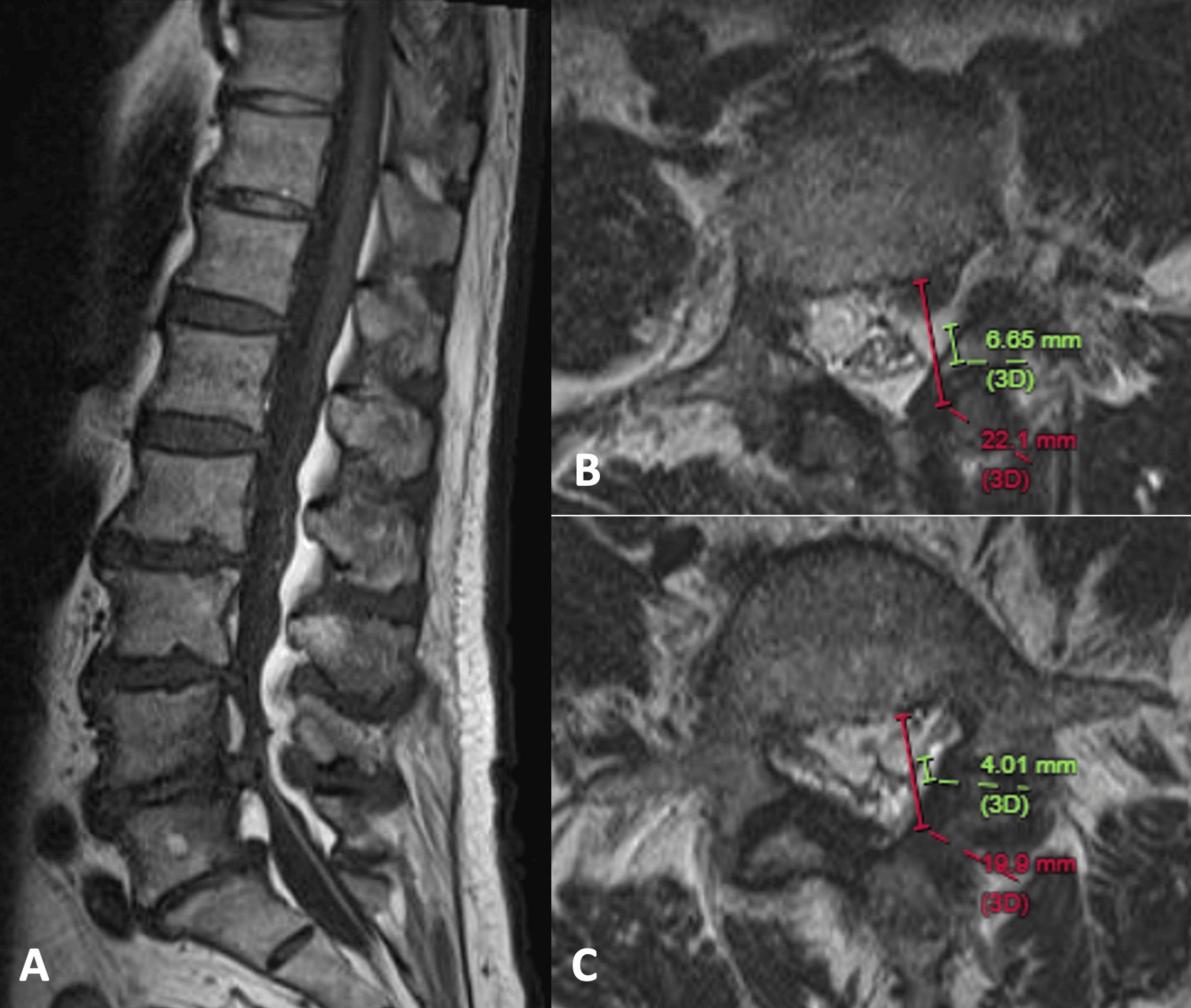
MRI of the lumbosacral spine of a 72-year-old man with chronic lower back pain and increasing spinal claudication. (A) Sagittal T1-weighted image shows excessive epidural fat, especially at the L3/4 to L5/S1 levels, both anterior and posterior to the dural sac. The combined effect of excessive epidural fat and degenerative changes results in severe spinal stenosis. (B) Axial T2-weighted image near the L3/4 level shows a polygonal dural sac (dural/fat ratio: 0.45, fat%: 70%), moderate SEL. (C) Axial T2-weighted image near the L4/5 level shows a stellate dural sac (dura/fat ratio: 0.25, fat%: 80%), severe SEL. MRI: magnetic resonance imaging; SEL: spinal epidural lipomatosis.
